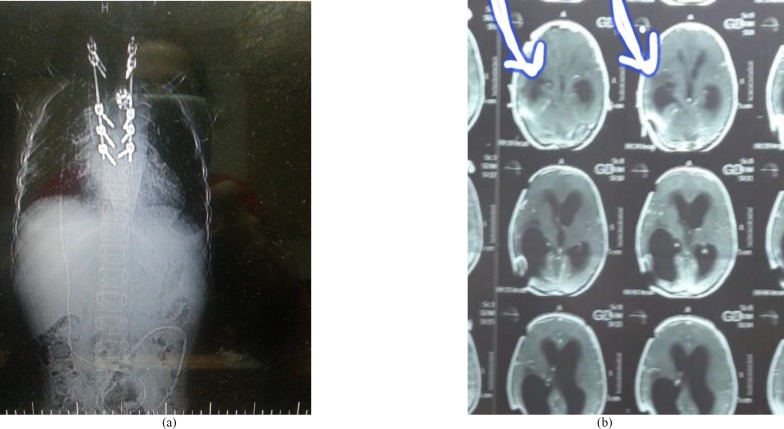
Imaging data in a chronic granulomatosis disease patient with invasive aspergillosis caused by *Aspergillus nidulans*; a) a plain X-ray revealing the laminectomy of L4-L5 with pedicular screw, along with post-operative changes, and exhibiting a destructive soft tissue density mass lesion centered on the body of T4-T5 vertebrae with adjacent prevertebral soft tissue formation mostly in the right side leading to central spinal column involvement and right fifth rib destruction, b) a computed tomography scan revealing brain abscess leading to hydrocephaly, showing extensive vasogenic edema in the right tempoparietal lobe, along with the dilations of the left ventricle and occipital horn of the right ventricle
